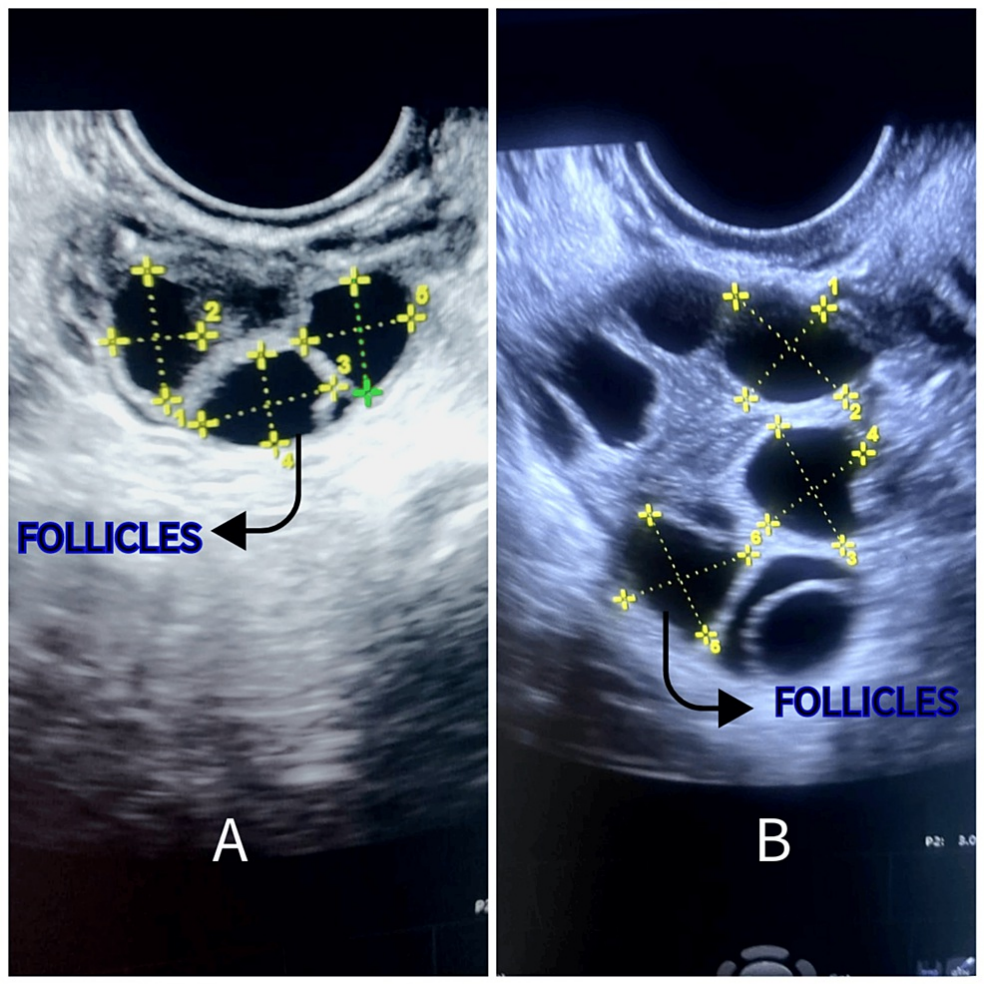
Ultrasonographic image of the right ovary showing follicles. (A) Before PRP therapy. (B) After PRP therapy. The original picture was captured by the author of this article, and informed consent was obtained for publication from the patient. PRP, platelet-rich plasma
